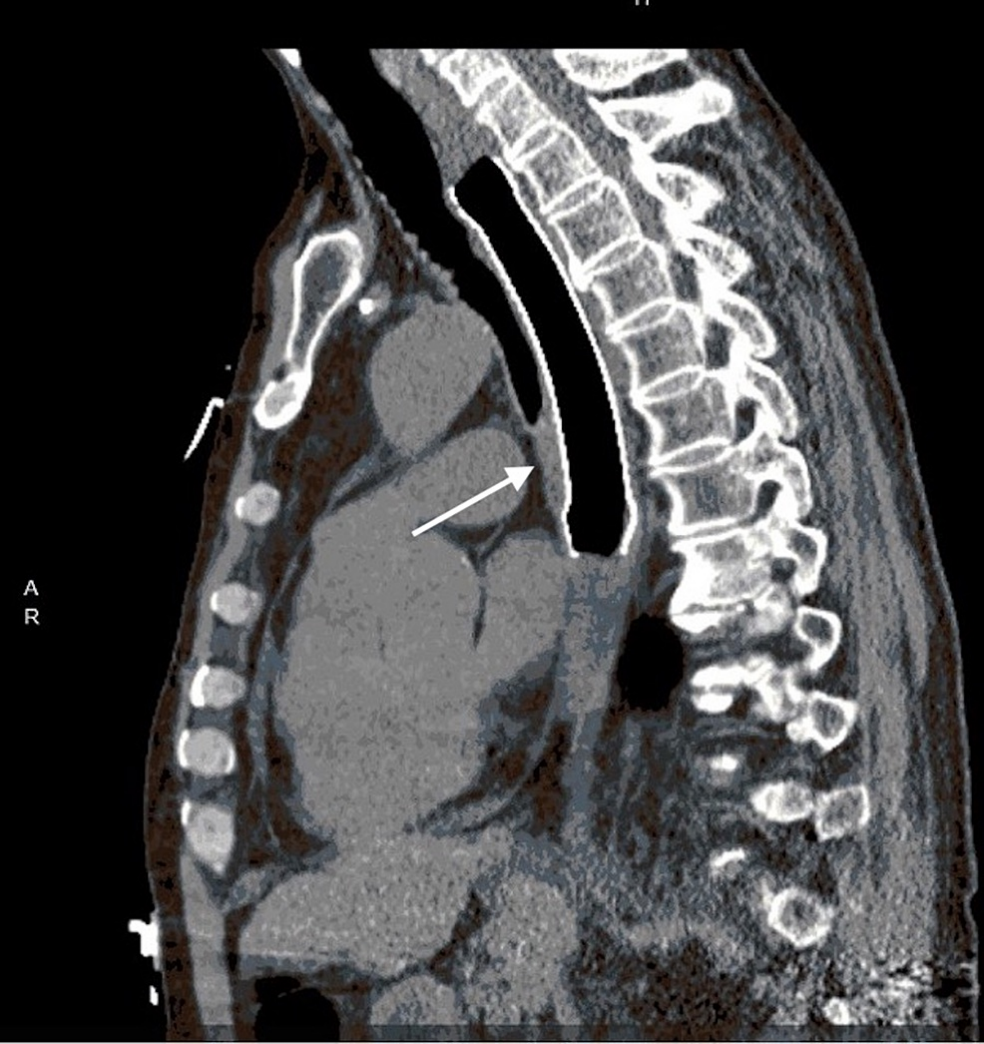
Coronal view of computed tomography image of the parenchymal-esophageal fistula after it was successfully closed by fully covered esophageal stent (arrow)
